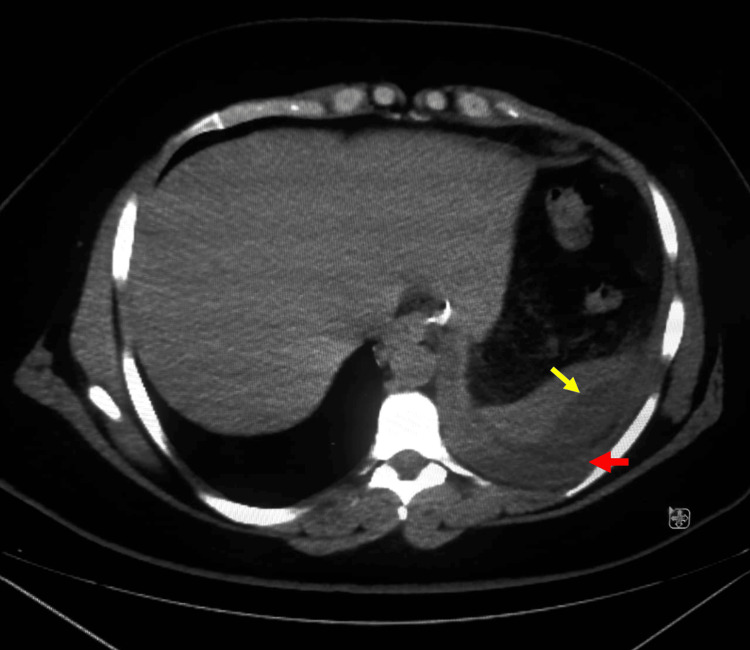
Contrast-enhanced abdominal CT in the axial plane taken two weeks after laparoscopic sleeve gastrectomy showed a splenic hematoma (yellow arrow) accompanied by a mild left-sided pleural effusion (red arrow).
